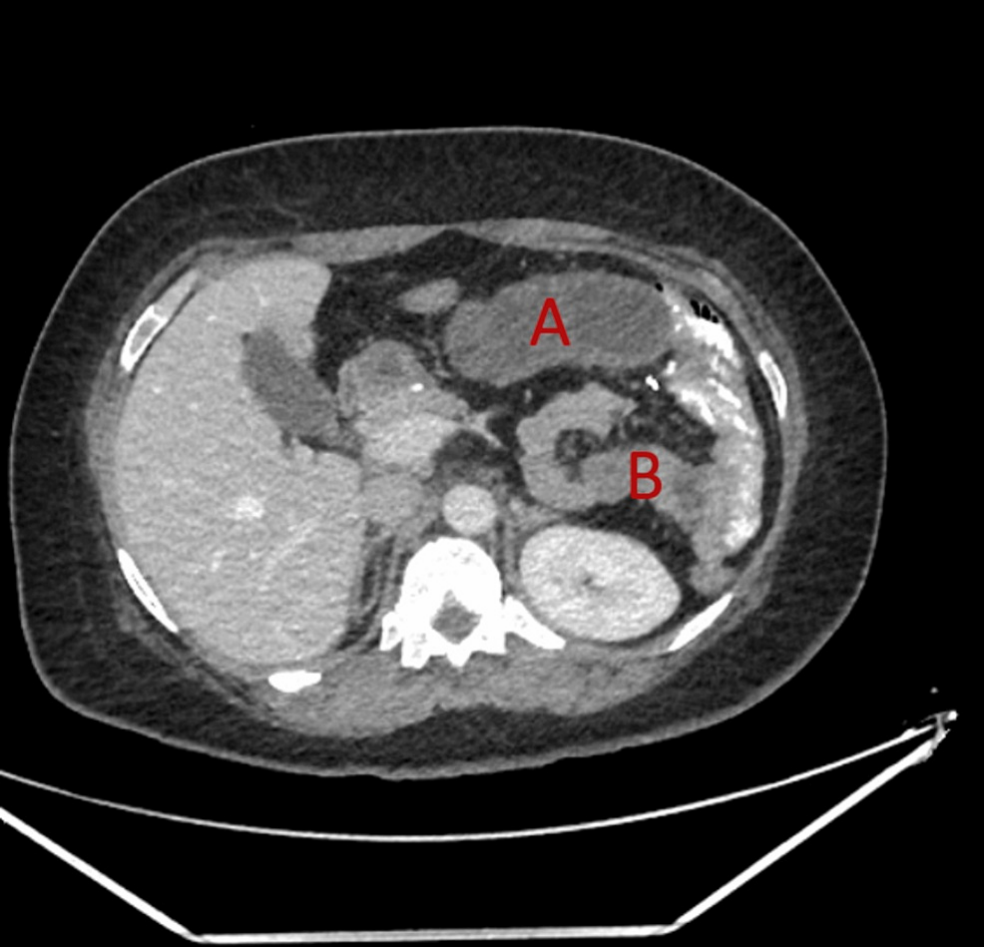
CT abdomen with contrast (axial view) showing the difference between (A) the proximal dilated part of the small intestine and (B) the distal collapsed slightly enhanced part (indicating signs of ischemia)
